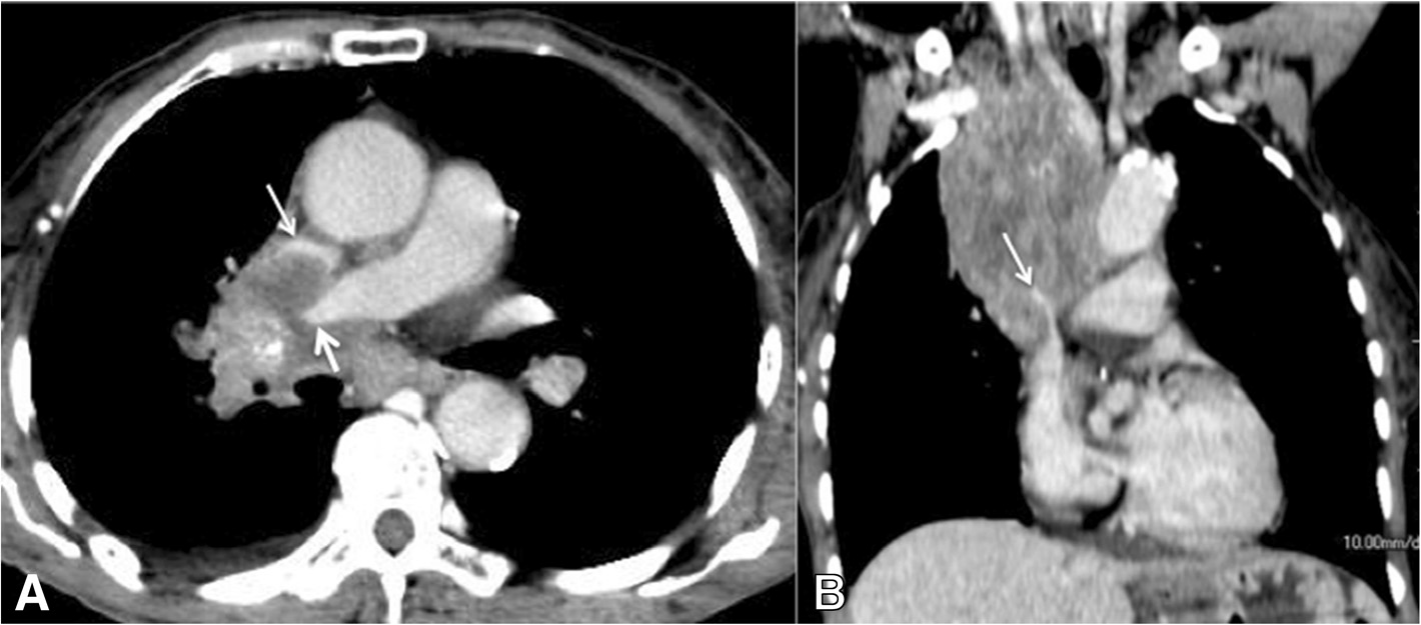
A 55-year-old man with superior vena cava syndrome. Computed tomographic images of a patient with metastatic laryngeal cancer showing an infiltrative mediastinal mass causing compression of the right pulmonary artery (thick arrow) and superior vena cava (thin arrow). (A) Post-contrast axial slice. (B) Post-contrast coronal reconstruction.
